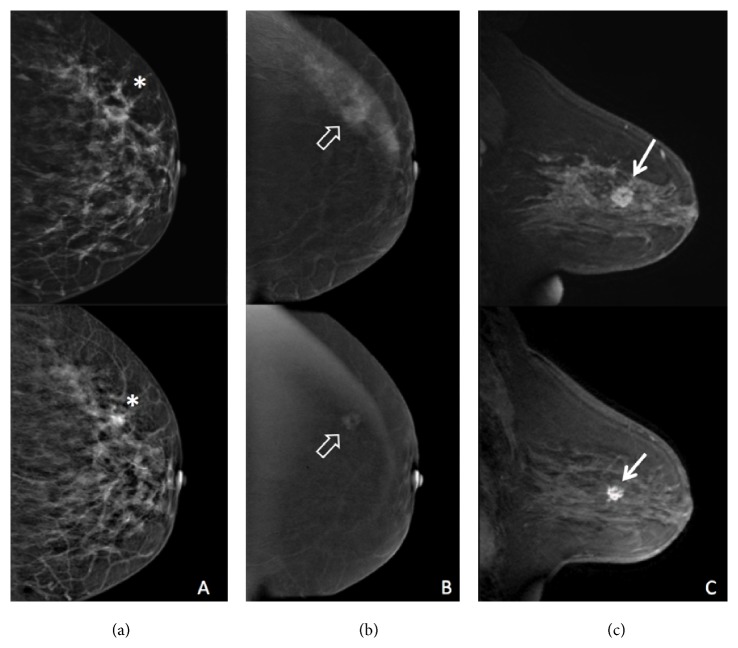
42-year-old woman. Invasive ductal carcinoma after neoadjuvant chemotherapy with residual disease. Pre-NAC (top row) and post-NAC (bottom row) FFDM (a), CEM (b), and MRI (c). A mass with distortion calcifications can be seen on FFDM (*∗*). Mass enhancement with irregular margins is shown on CEM (open arrow) and MRI (arrow).
